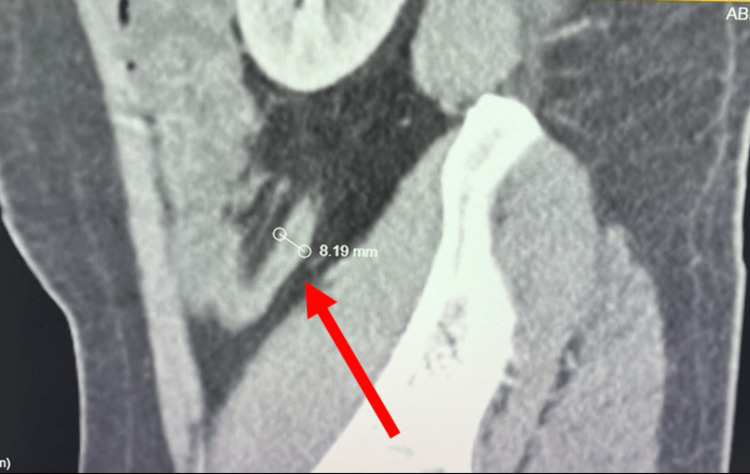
Contrast-enhanced CT of the abdomen demonstrating appendiceal enlargement. Sagittal contrast-enhanced CT image of the abdomen demonstrating an enlarged appendix measuring approximately 8.2 mm in diameter (arrow), consistent with acute appendicitis.
